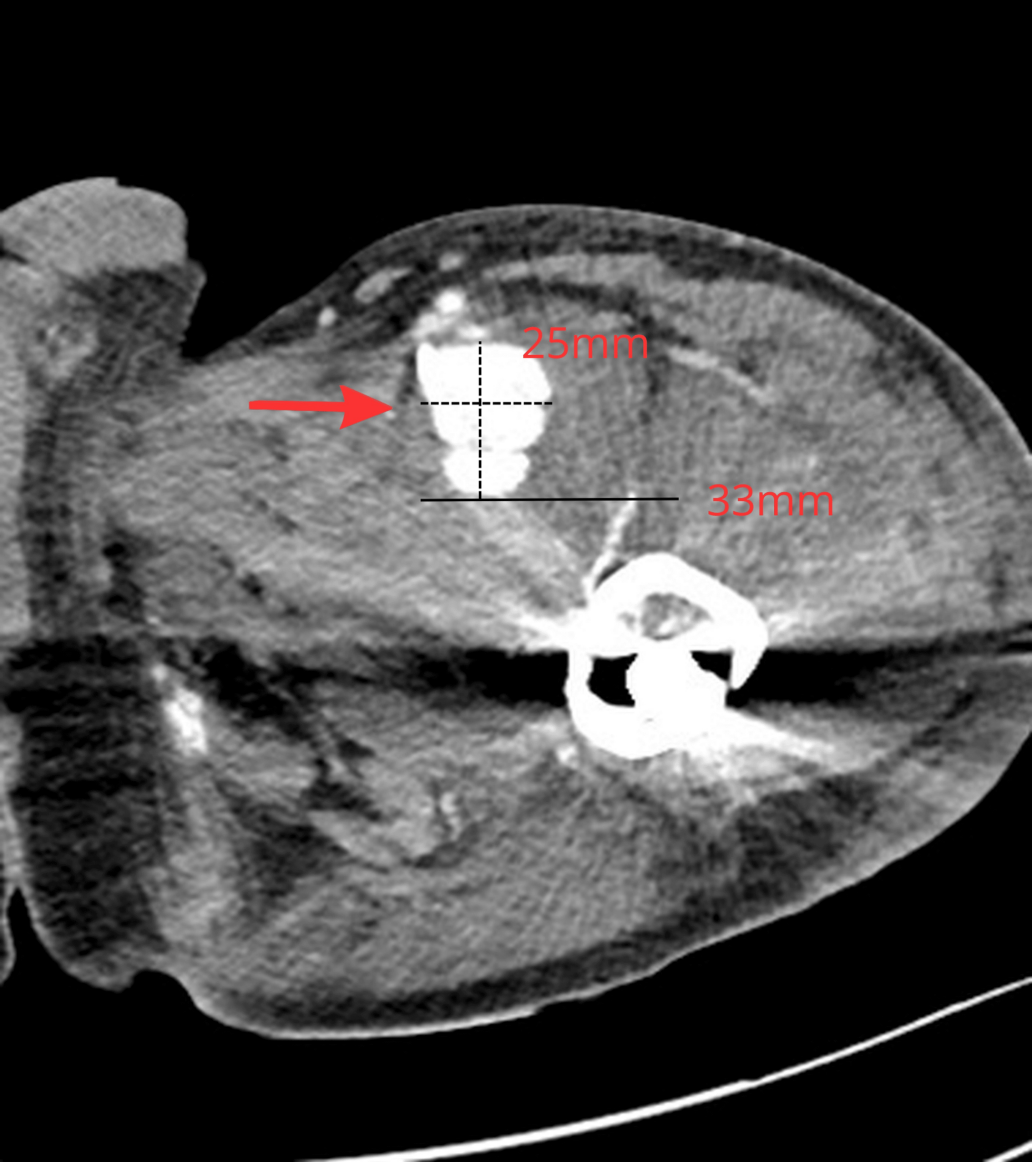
CT angiography demonstrating a pseudoaneurysm of the left PFA. No vascular abnormality was present on a PET-CT scan performed 3 weeks prior to the traumatic event. The pseudoaneurysm measures approximately 25 mm in maximal diameter and is in direct spatial contact with the displaced lesser trochanter fragment, with a measured distance of approximately 33 mm.
